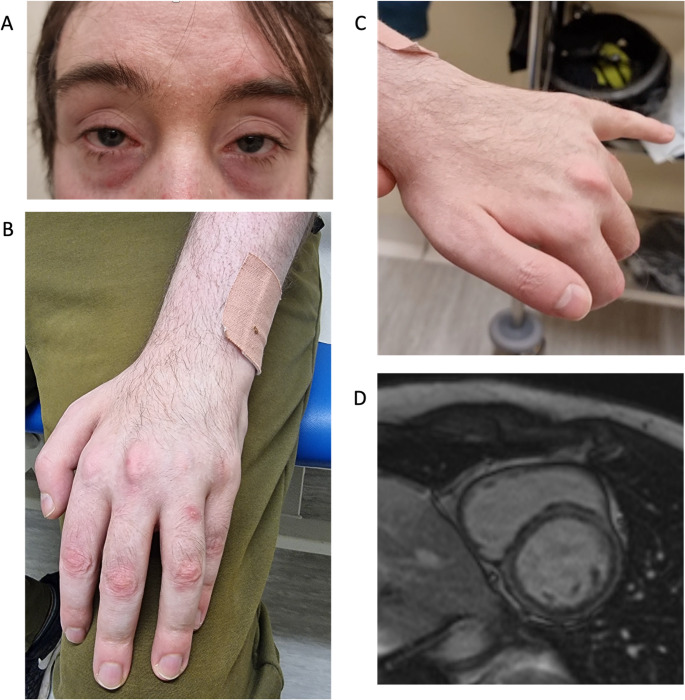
Clinical features of *PLEC* CMS. (a) Bilateral ptosis (fatiguable); (b, c) Upper limb weakness, wasting of intrinsic hand muscles, and finger and wrist drop (also fatiguable) mimicking slow channel CMS; (d) Late gadolinium enhancement cardiac magnetic resonance imaging demonstrating a non-ischaemic pattern of myocardial fibrosis with ring-like enhancement at basal and mid-ventricular levels. Informed consent was obtained for the publication of images
